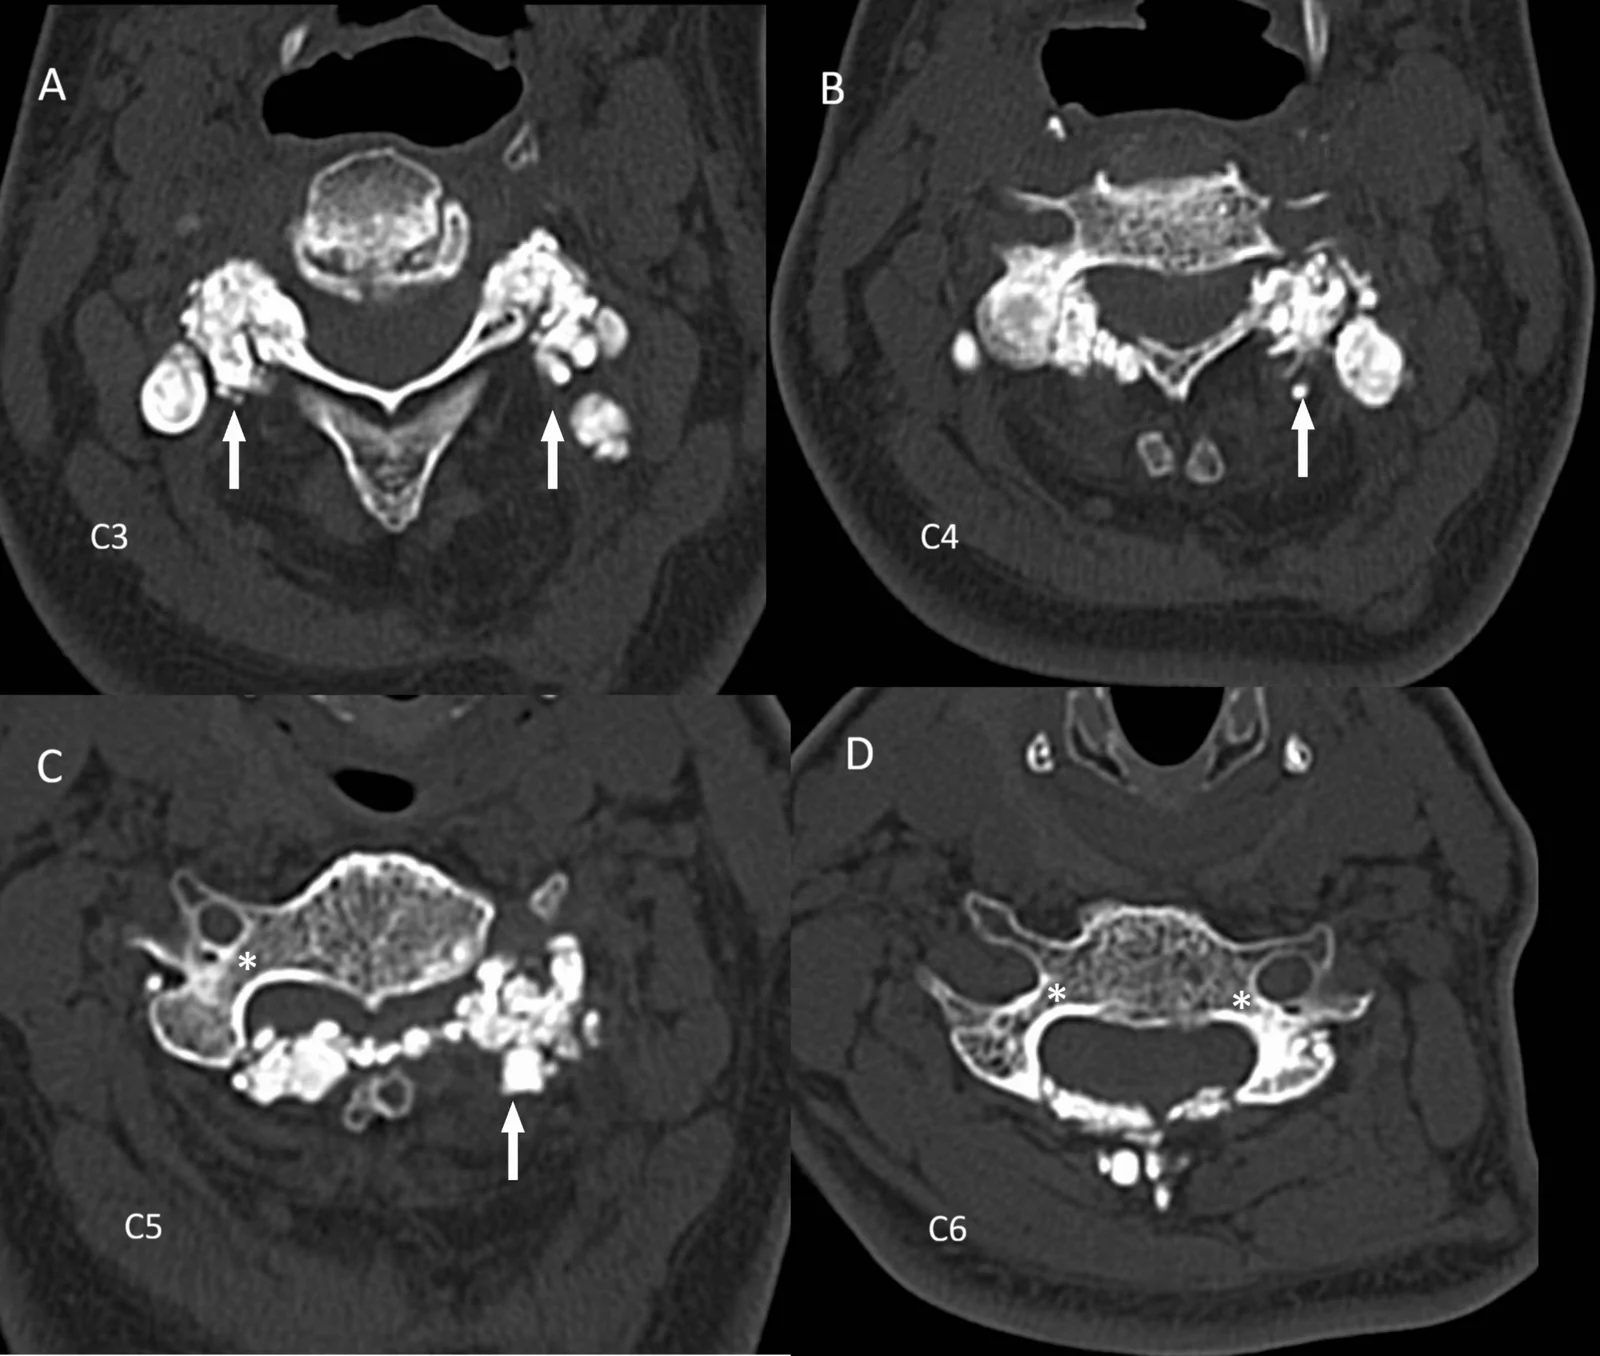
Representative axial cuts of cervical spine CT: (A and B) extensive destruction of both C3 and C4 lateral masses (arrows). (C) Destruction of the left C5 lateral mass (arrow) with an intact right pedicle (asterisk). (D) Replacement of C6 lamina with calcinosis with intact pedicles on both sides (asterisks).
